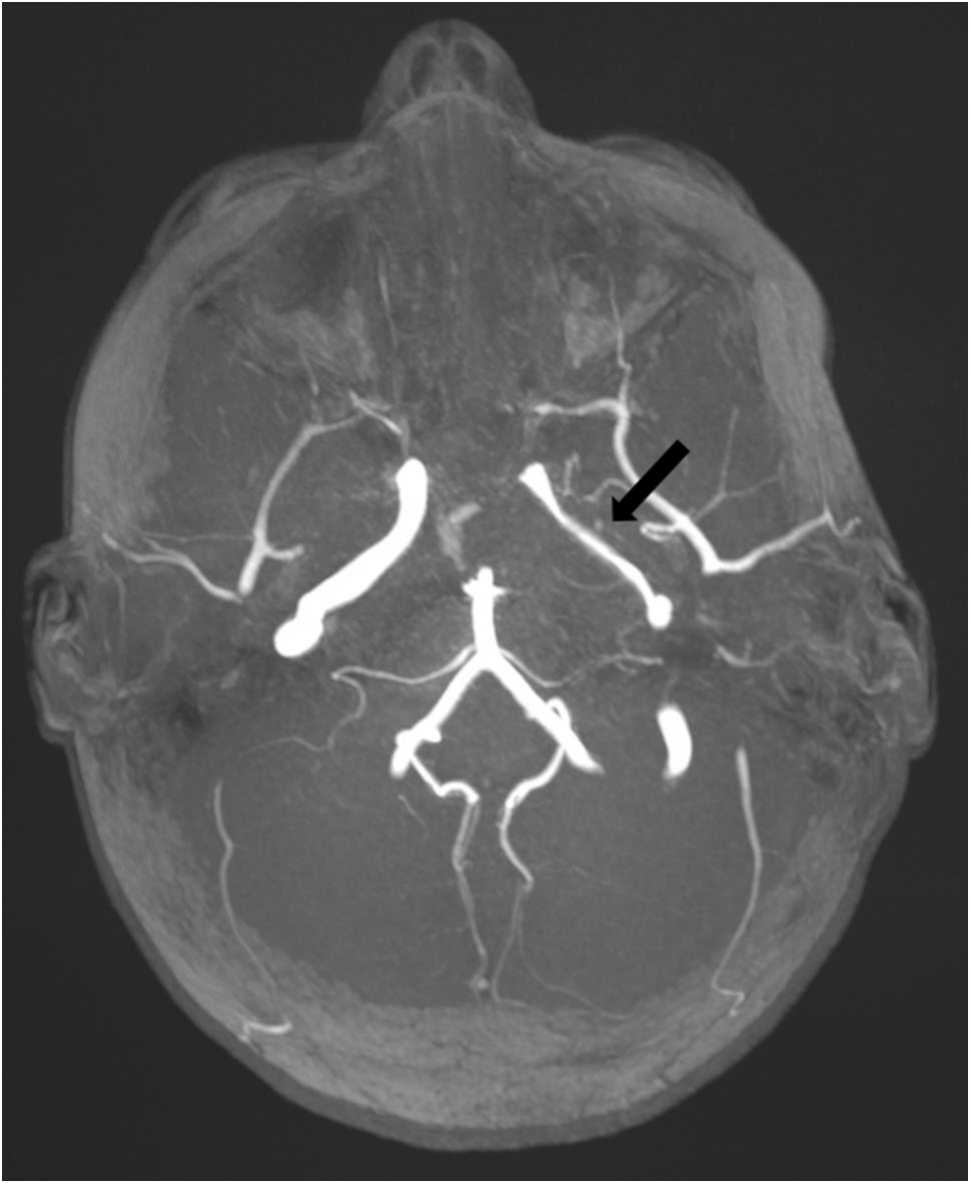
Axial multi-slab of a time-of-flight angiography 3-dimensional sequence magnetic resonance image without contrast agent administration in a 5-year-old girl with extensive abscess at the left skull base with reactive vasculitis of the internal carotid artery in the petrous part M1 (*arrow*) as a complication of otitis media and mastoiditis
